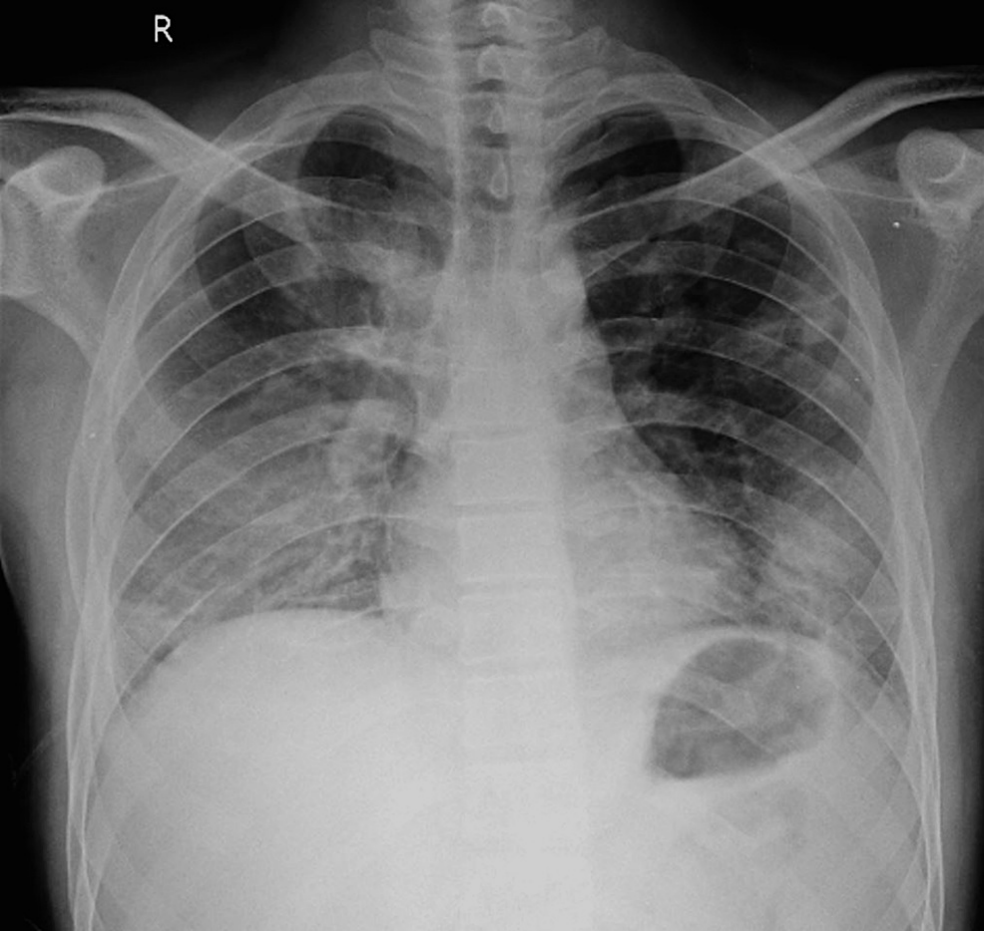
Chest x-ray posteroanterior (PA) view, post-thoracic pigtail catheter insertion on the right side, showing improvement in the right-sided pleural effusion
